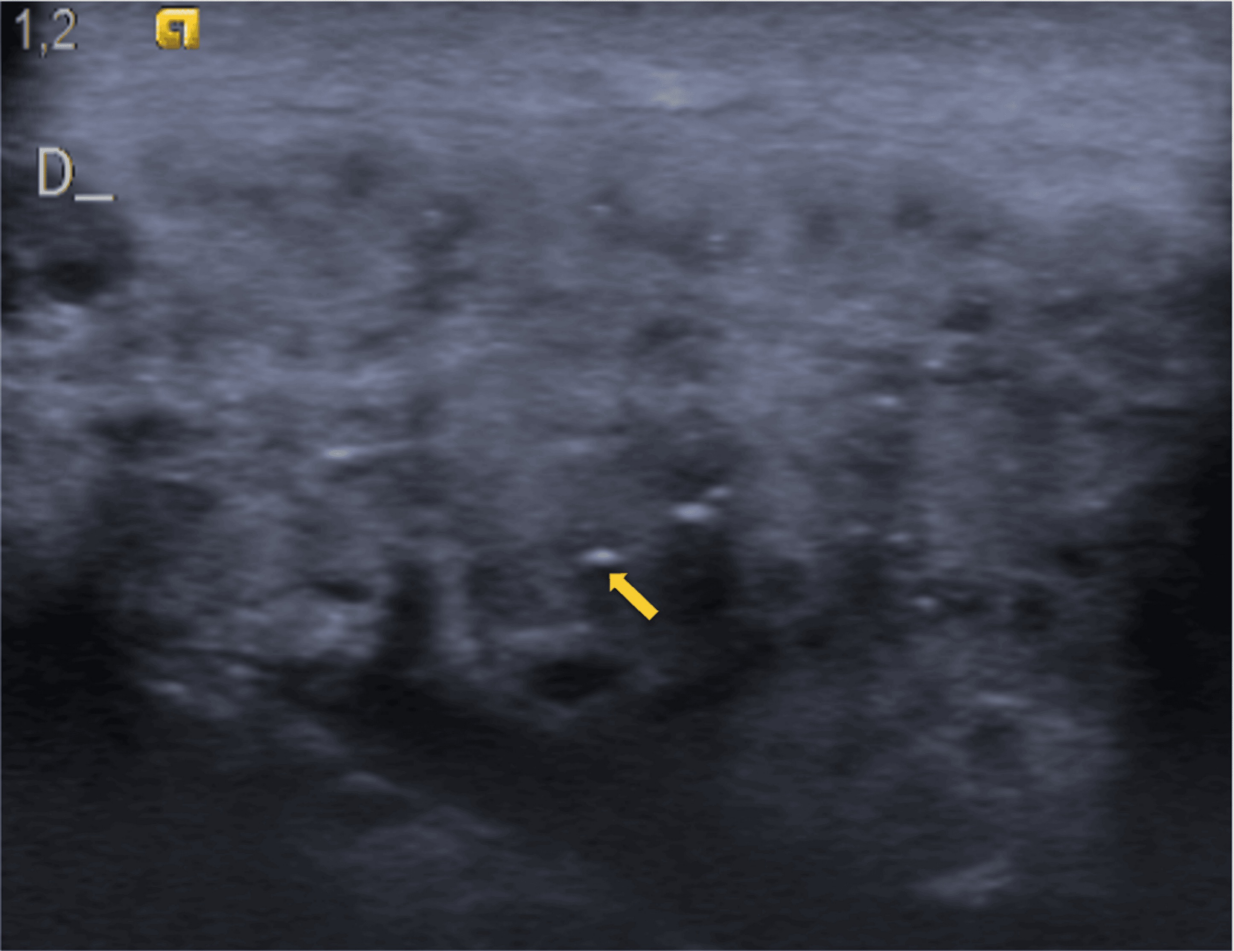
Ultrasound Ultrasound image of the right parotid gland, which appears enlarged and heterogeneous, shows multiple anechoic spaces corresponding to ductal branches, containing echogenic foci (arrow) that represent gas bubbles.
